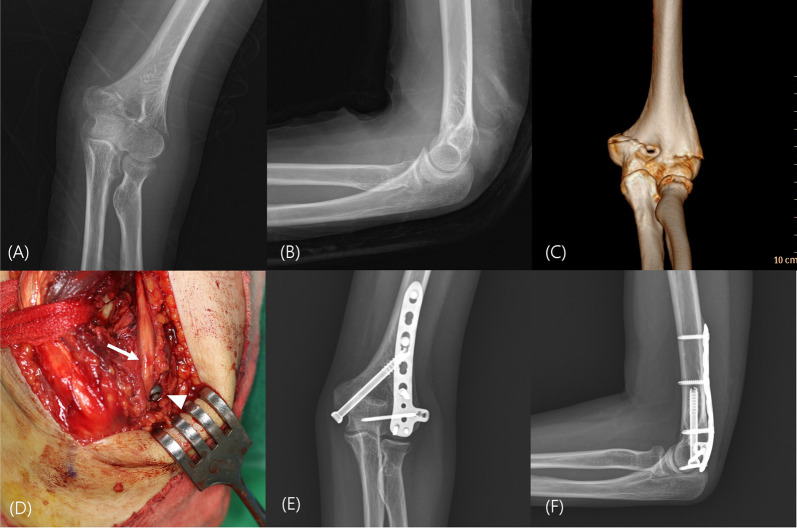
A 29-year-old female patient treated with single-plate fixation with a medial screw. A, B Preoperative radiographs showed an extra-articular distal humerus fracture. C Three-dimensional computed tomography reconstruction of the fractured distal humerus. D The medial cannulated screw (arrow head) was positioned anterior to the ulnar nerve (arrow). E, F Postoperative radiographs obtained at final follow-up demonstrated bony union
